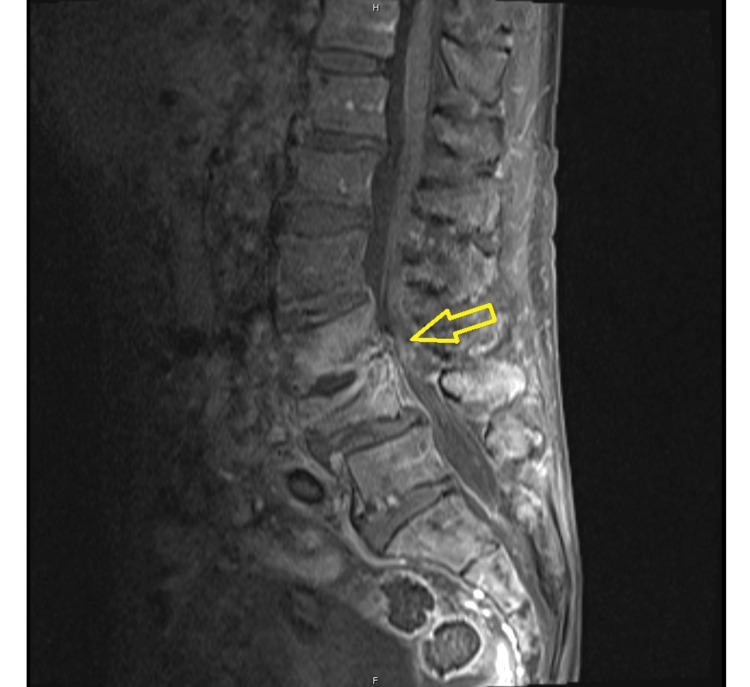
MRI of the lumbar spine, sagittal view Arrow highlights collapse of superior endplate of the L4 vertebra. There is prominent enhancement of the vertebral bodies with enhancing paraspinal granulation tissue, as marked with the arrow head.
